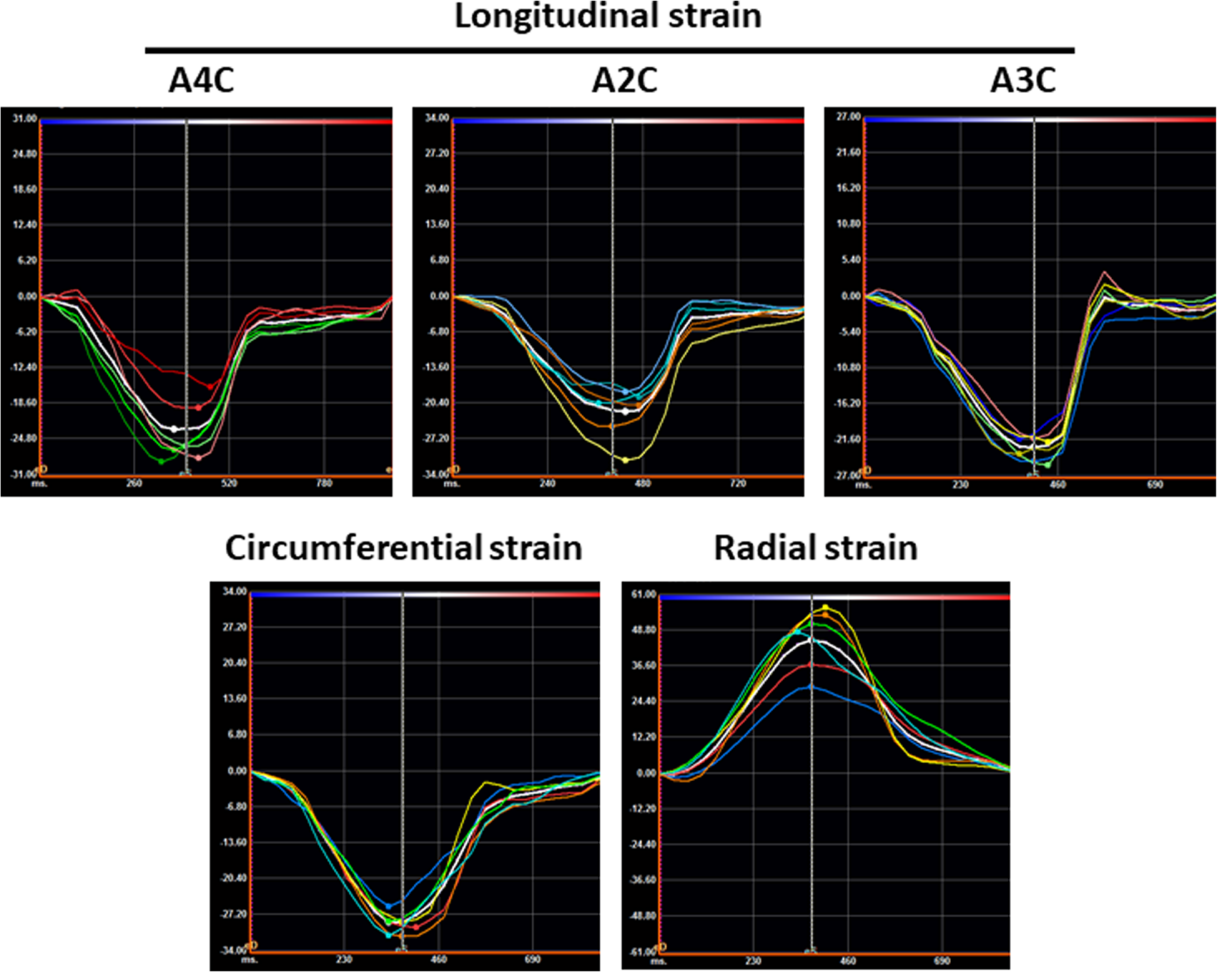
Left ventricular strain curves. Average and segmental longitudinal strain curves were obtained from the apical 4-chamber (A4C), 2-chamber (A2C), and 3-chamber (A3C) views. Average and segmental circumferential and radial strain curves were obtained from the parasternal long-axis view at the level of the papillary muscle. Global longitudinal and circumferential strain measurements were derived from the nadir of the average longitudinal and circumferential strain curves respectively. Global radial strain measurements were derived from the peak of the average radial strain curve
